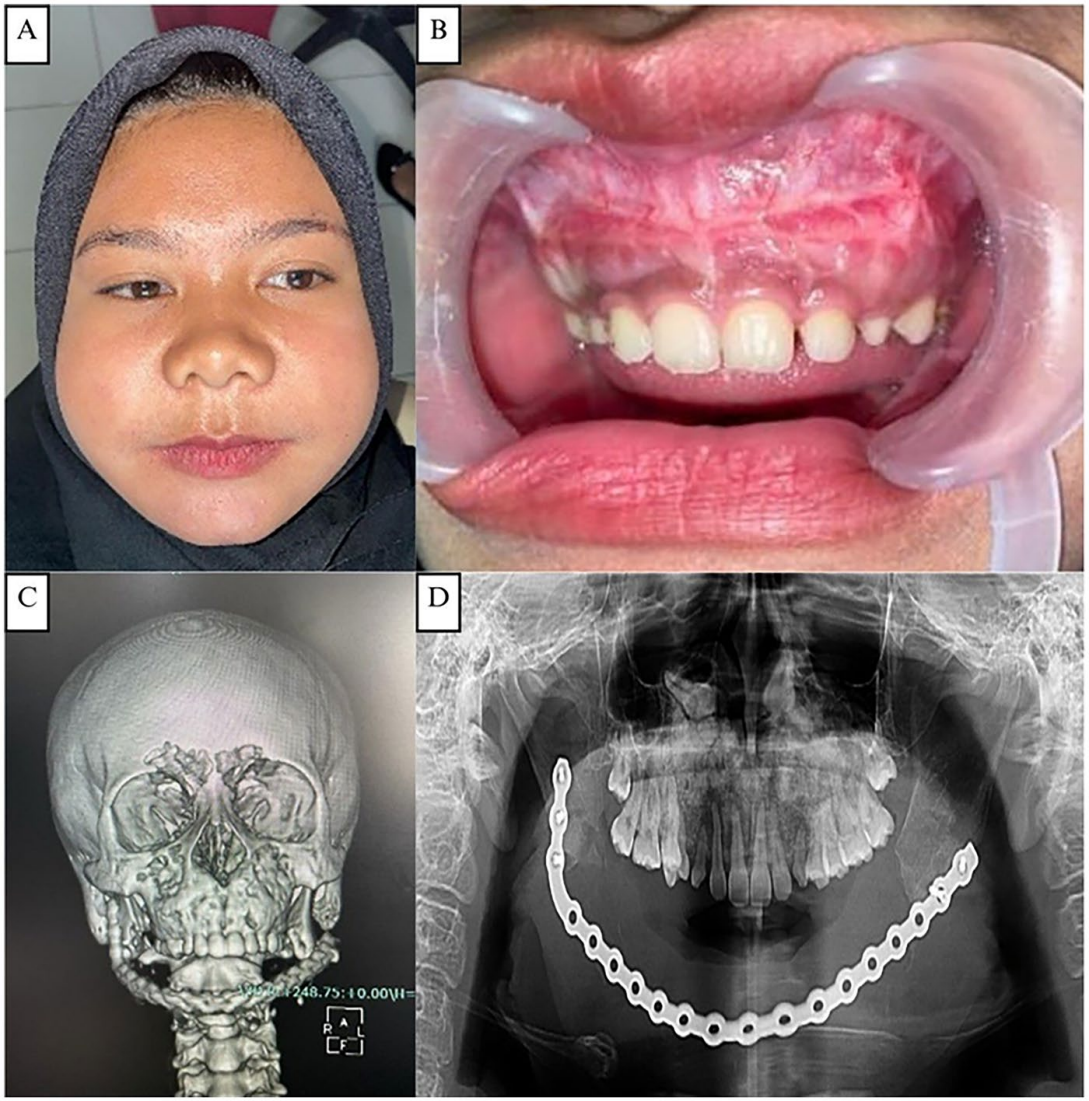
(A) The fourth case, a patient after 2 years follow up complaint with swelling in the maxillary area. (B) Pre-operative intraoral clinical appearance. (C) The CT scan showed a solid mass accompanied by calcification in the maxillary bones without soft tissue infiltration. (D) OPG showing an internal fixation installed with a bridging plate and screws, which fixed the mandibular corpus defect.
